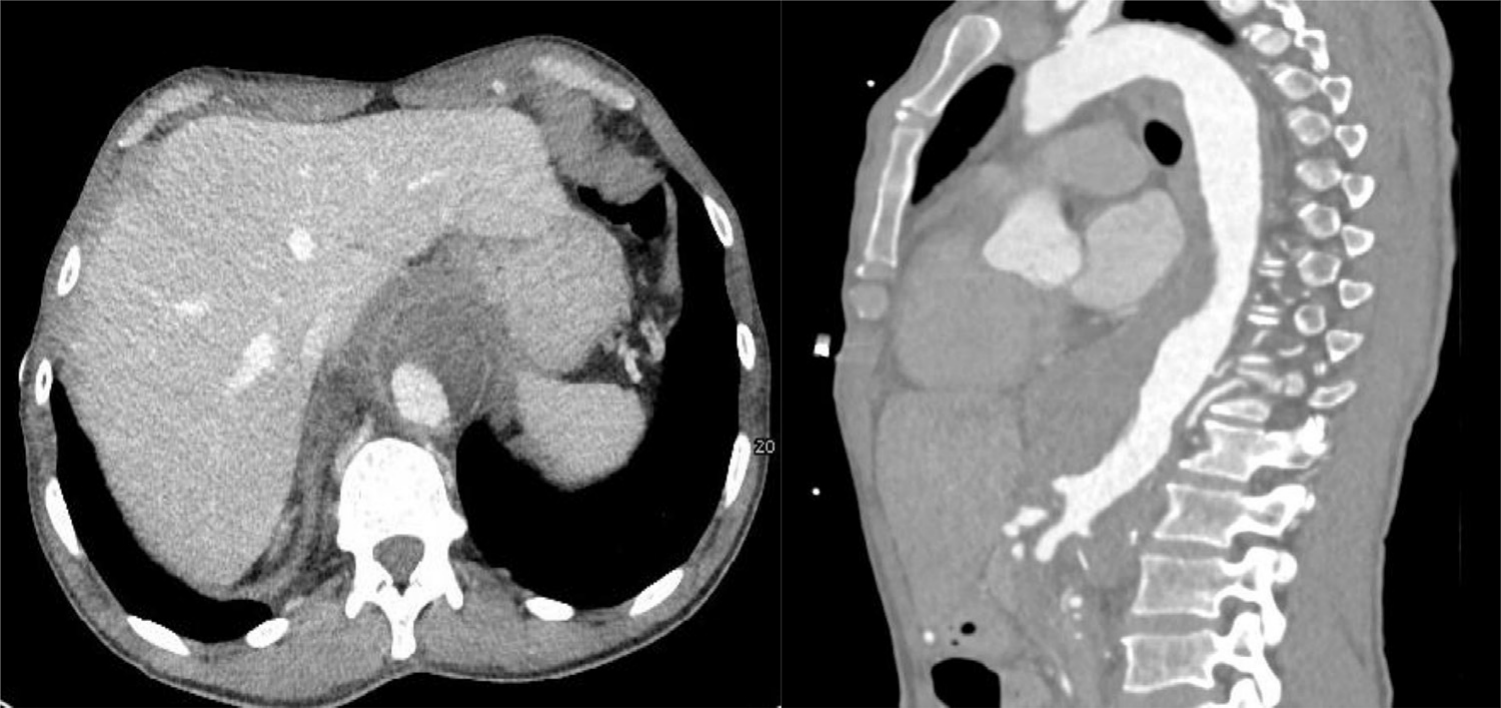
CTA showing lobulated thoracoabdominal aneurysm with periaortic inflammatory changes, soft-tissue cuff, and lymph node manchette, consistent with suspected mycotic aneurysm.
